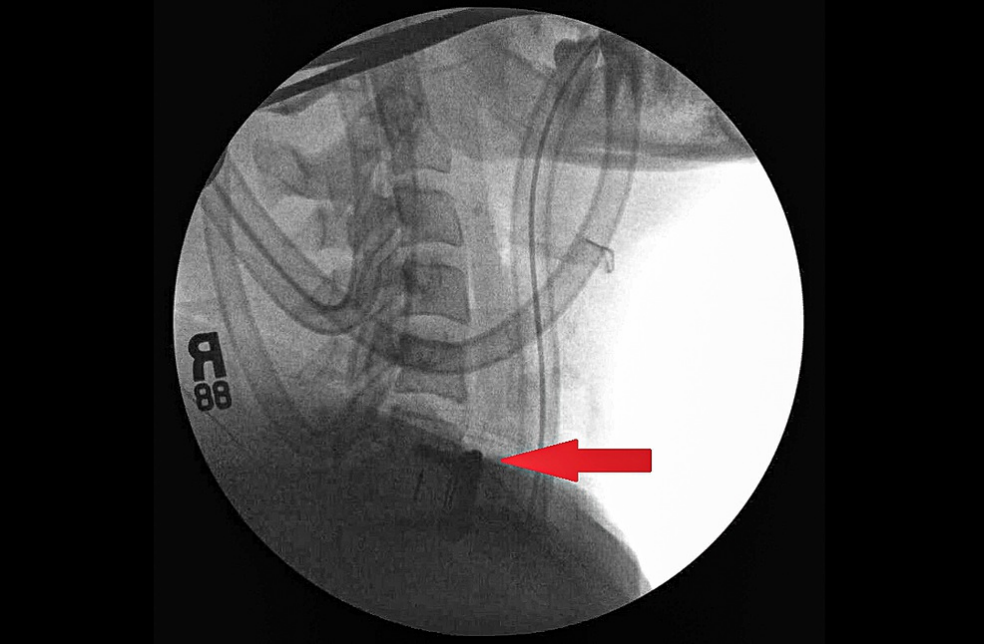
X-ray with fluoro imaging of anterior cervical fusion at C6-C7.
